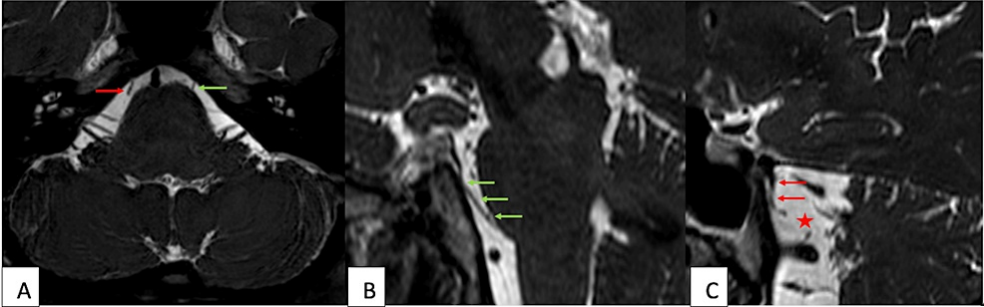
Axial (A) and sagittal oblique (B, C) 3D T2-weighted images of the posterior fossa. Panels A and B demonstrate the usual course of the intact left CN VI from the brainstem through the cisternal segment into Dorello’s canal (green arrows). In Panels A and C, the proximal cisternal segment of the right CN VI (red arrows) is not seen, and the distal cisternal segment is thickened, redundant, and discontinuous with the brainstem. A red star indicates the gap between the visualized cisternal segment and the brainstem. CN VI: Sixth cranial nerve.
